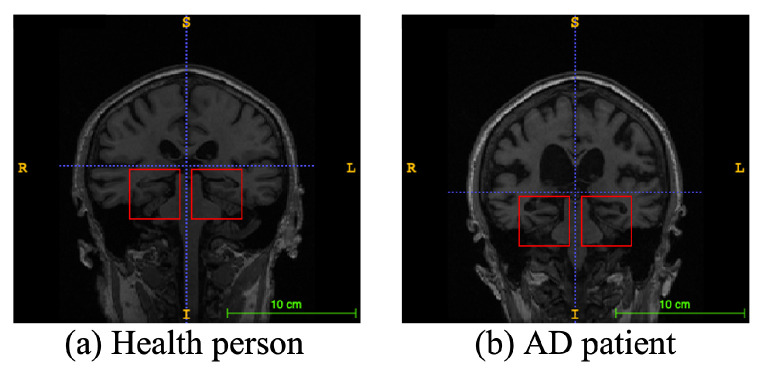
An example of different types of magnetic resonance images. The left image is a healthy person, and the right one is an Alzheimer’s disease patient. The red boxes represent hippocampi.
